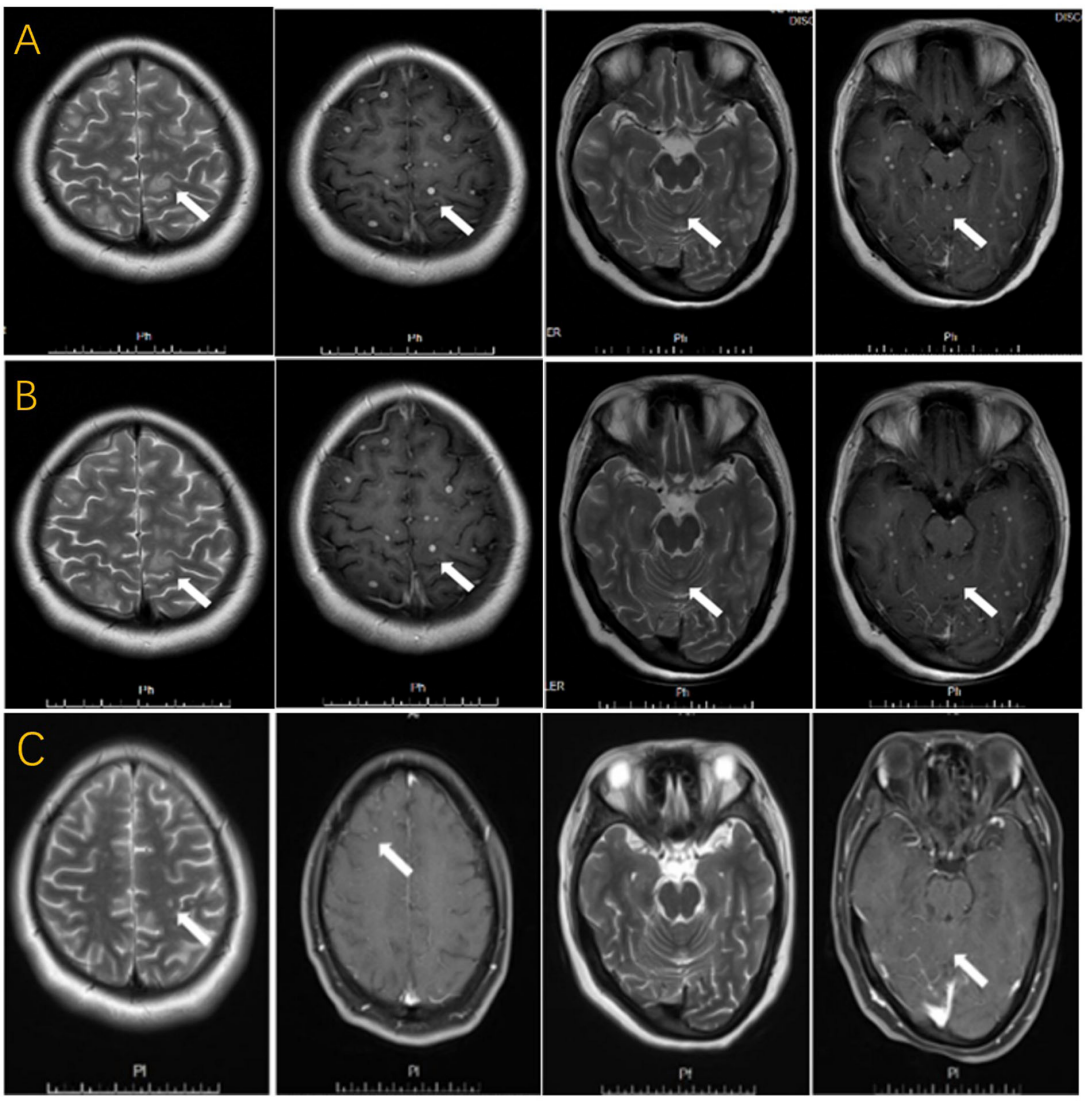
(A) T2-weighted image with multiple patchy hyper-intensity signals and “target sign”. T1-weighted post-gadolinium contrast image with multiple nodular enhancing lesions, similar to military tuberculosis. (B) T2-weighted image and T1-weighted post-gadolinium contrast image done after regular ATT for a month with no significant improvement. (C) T2-weighted image and T1-weighted post-gadolinium contrast image done after intrathecal injections for one month with significant decreased lesions.
